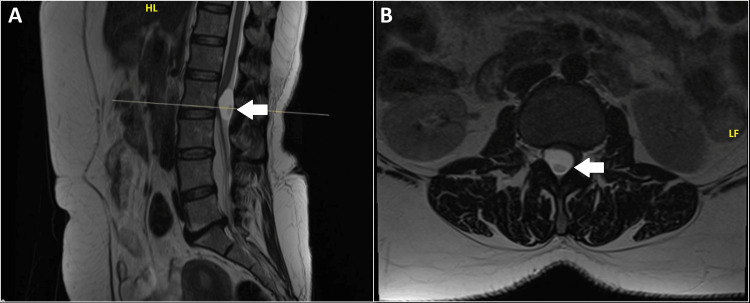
Sagittal (A) and axial (B) contrast-enhanced MRI views of the lumbar spine show a well-defined, ovoid, non-enhancing extra-medullary intradural lesion at the level of the L2 vertebral body. Arrows in the images indicate the lesion, which significantly compresses the conus medullaris and cauda equina roots, predominantly affecting the anterior and left side. HL: head-left, LF: left-hand.
